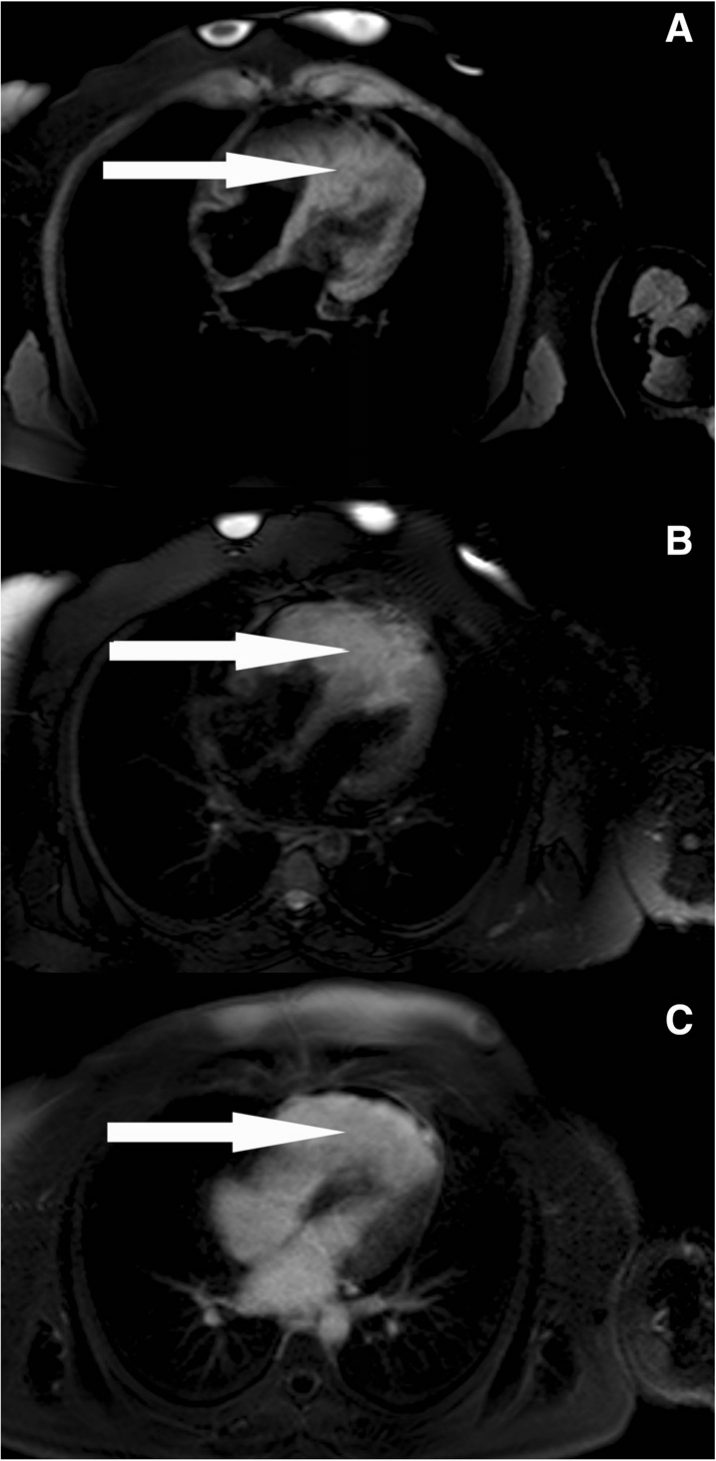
T1-weighted (a) and T2-weighted (b) images and late gadolinium enhancement (c) on cardiovascular magnetic resonance imaging
